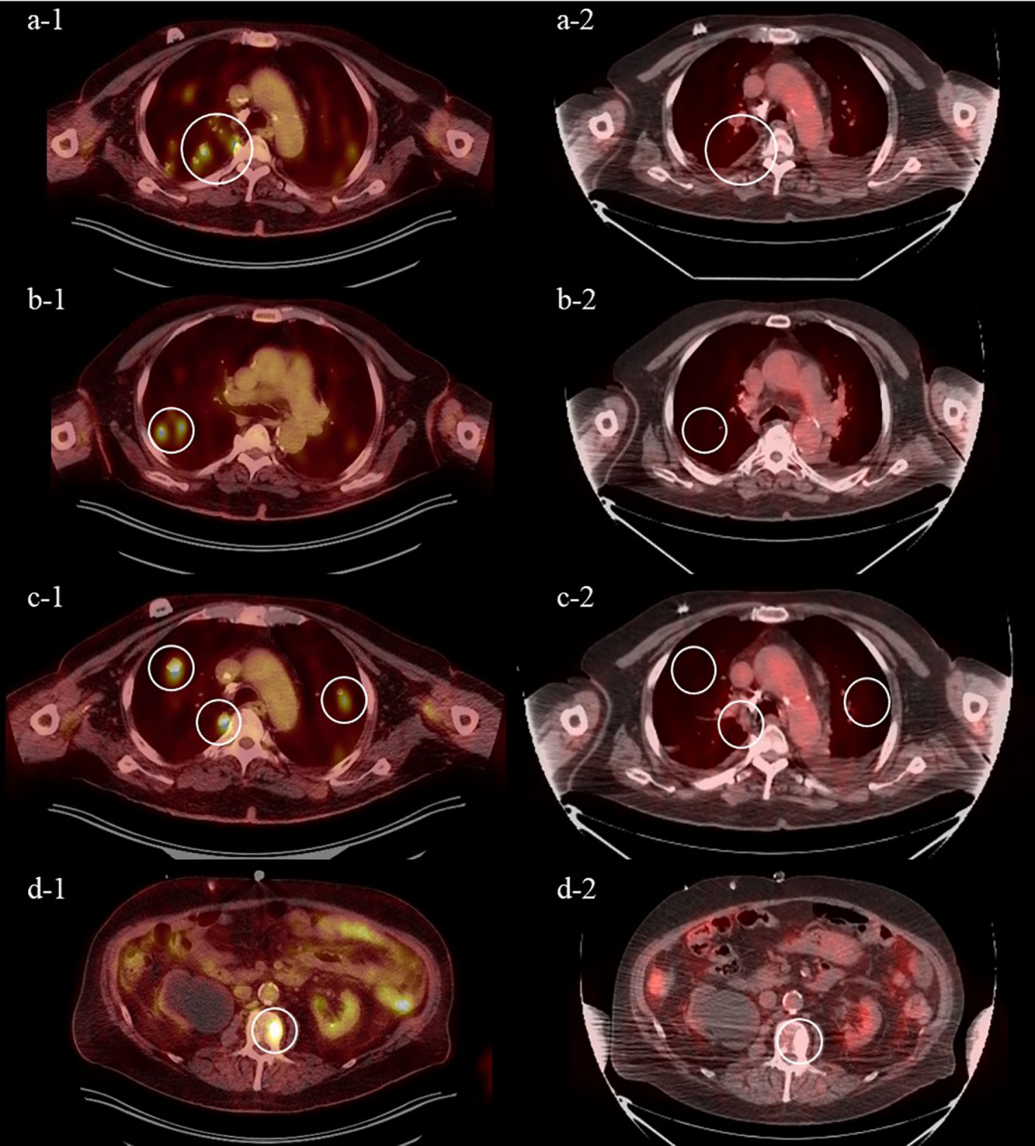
Positron emission tomography (PET) from August 2021 after 4 cycles of pembrolizumab demonstrating increase in size and number of FDG-avid pulmonary nodules (A-1–C-1) and FDG-avid sclerotic L2 lesion (D-1) and from November 2021 after 3 cycles of enfortumab vedotin demonstrating near complete response of FDG-avid pulmonary nodules (A-2–C-2) and FDG-avid L2 lesion (D-2).
